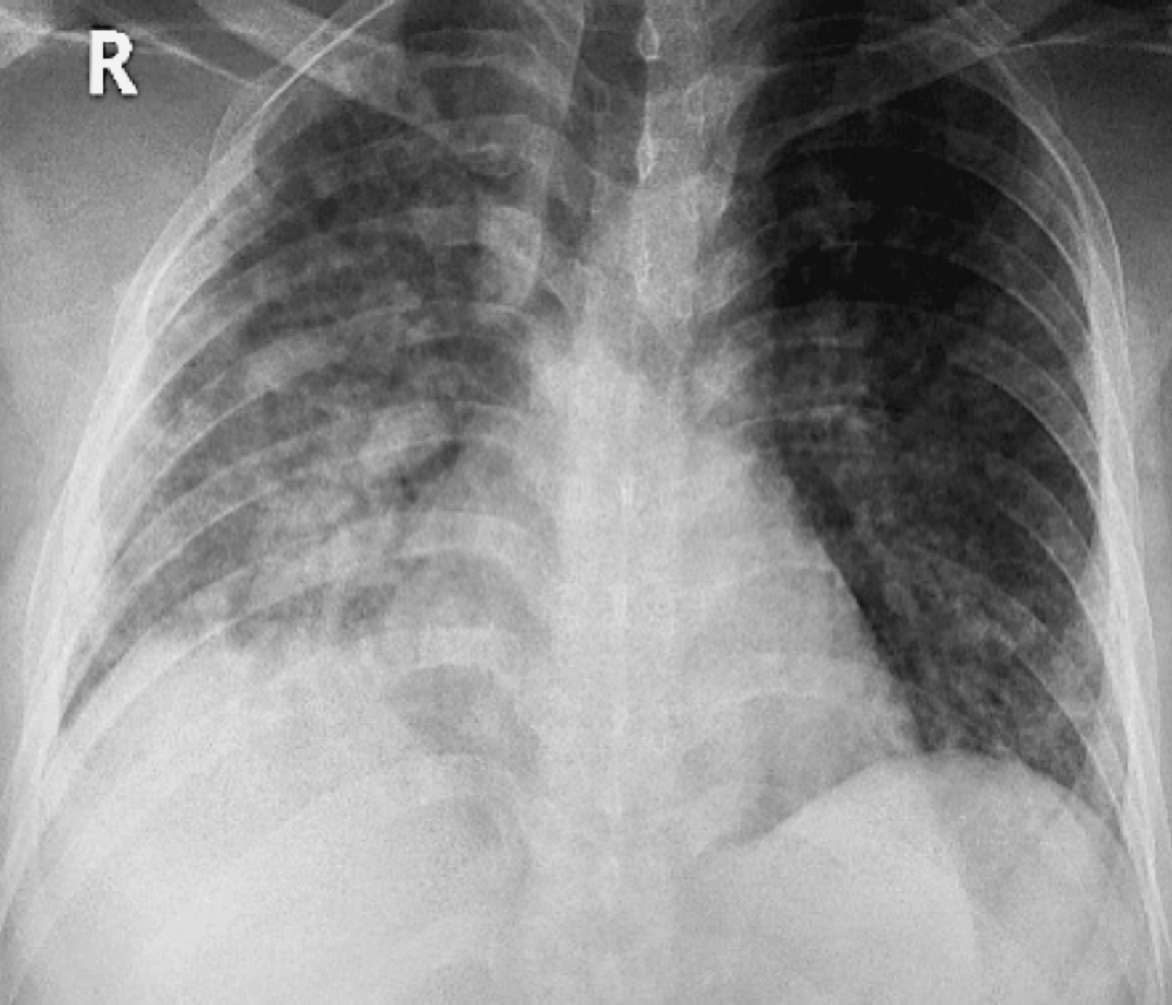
Chest radiograph of Case A on the day of presentation The image shows ground glass opacification with nodular infiltration seen in the right lung field
